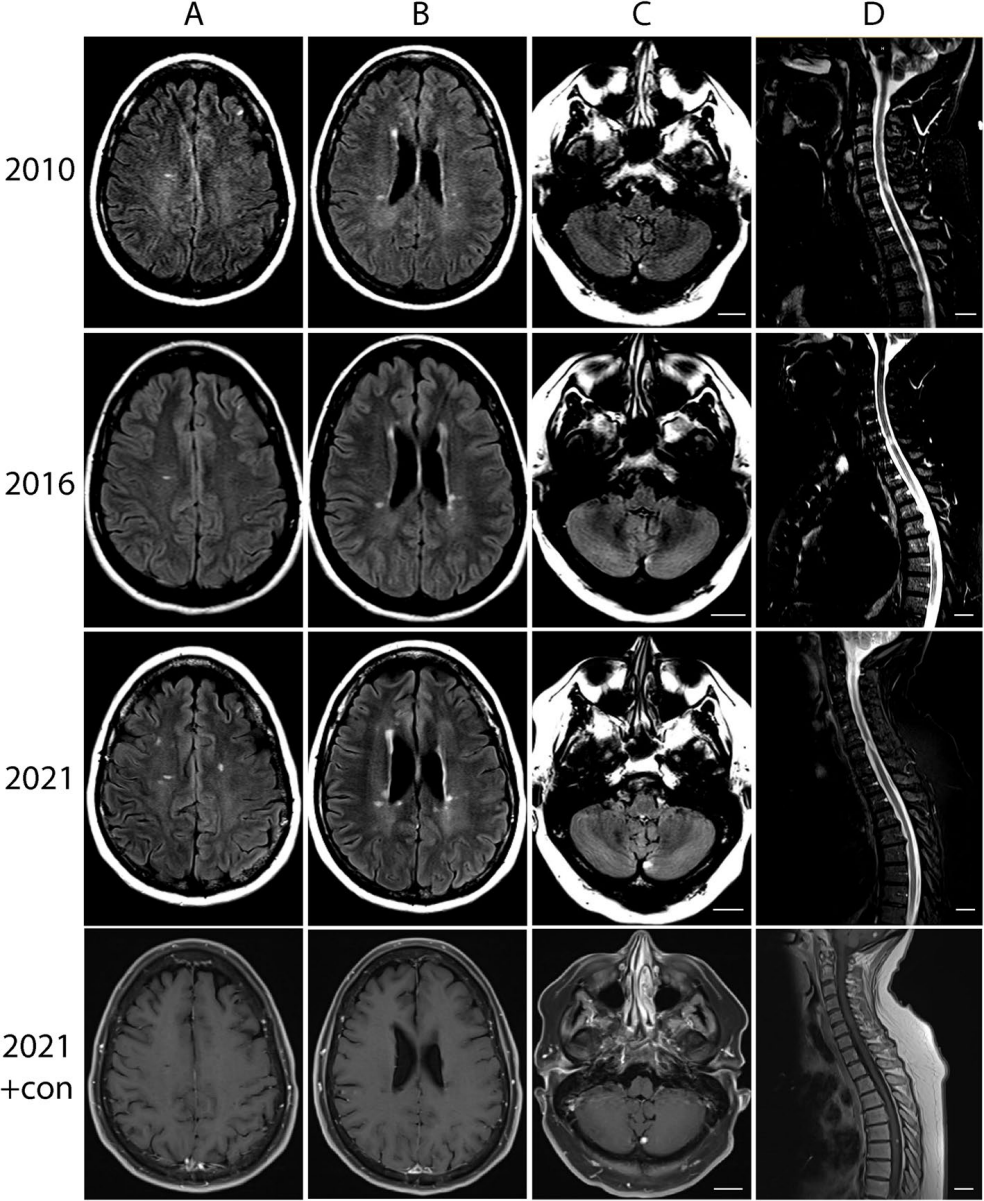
Serial MRI performed in 2010 (row 1), 2016 (row 2), and 2021 (row 3) showing stable cerebellar hemangioblastoma (panel C) and increasing burden of T2 FLAIR hyperintense lesions disseminating in time and space. STIR-weighted image of the spinal cord showing an enhancing lesion in the cervical cord (panel D). Row 4 depicts contrast enhanced T1 sequence of 2021 MRI
